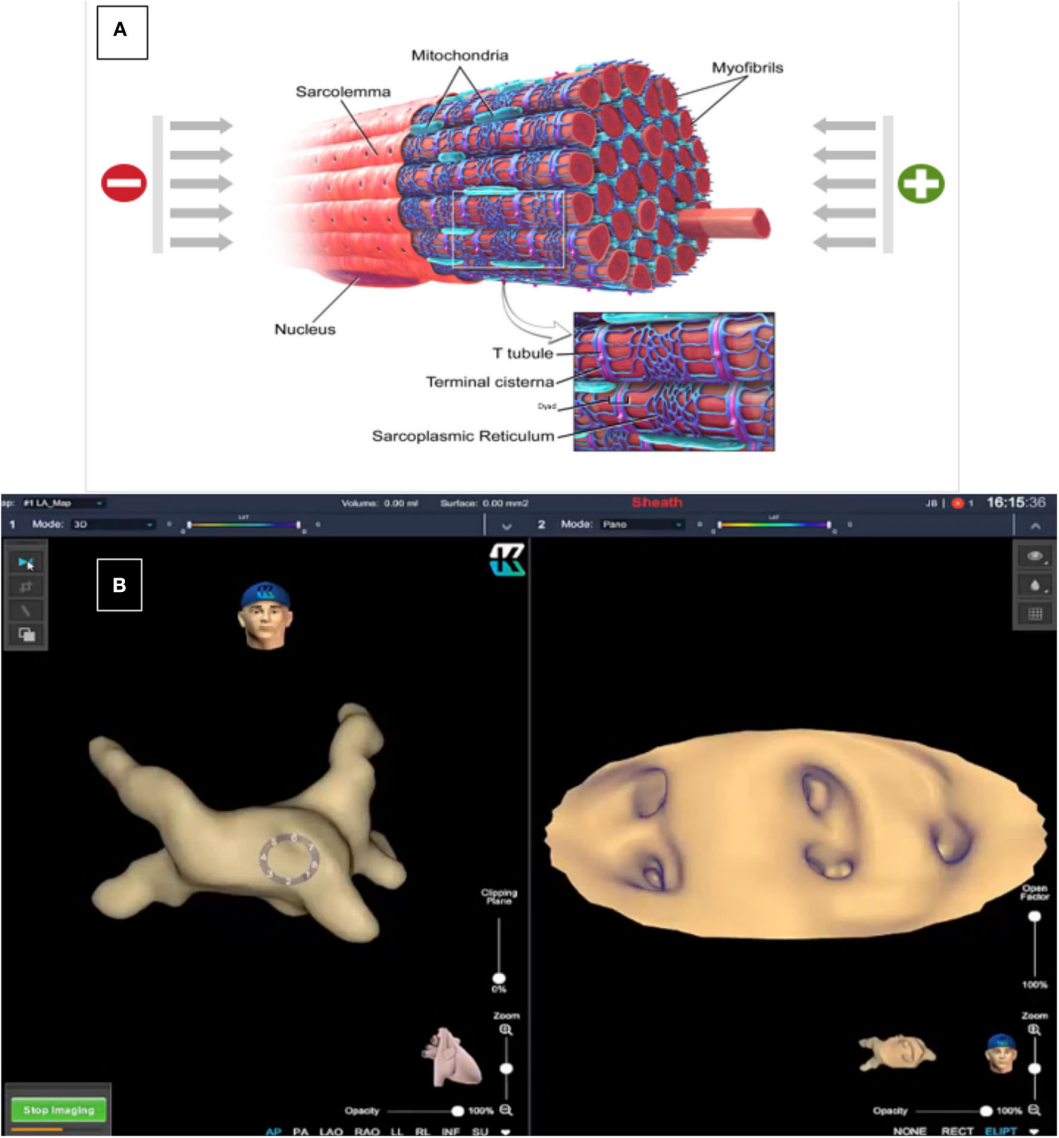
Dielectric Sensing Technology has the ability to differentiate material characteristics by measuring their dielectric coefficients. In the myocardium, electric charges can be conducted directly through the tissue (mainly through the intracellular fluid) but part of the charges will be stored (mainly in the sarcoplasmic reticulum that serves as a capacitor). These charges will be released after a short time. The conduction and capacitance values are frequency dependent and are affected by the state of the tissue (A). This technology permits 3-D reconstructions and CT-like endocardial views of the different cardiac tissues [(B), left: 3-D reconstruction of the left atrium; right: endocardial view of the four pulmonary veins and left atrial appendage ostia]. Image courtesy of EPD Solutions, a Philips company.
